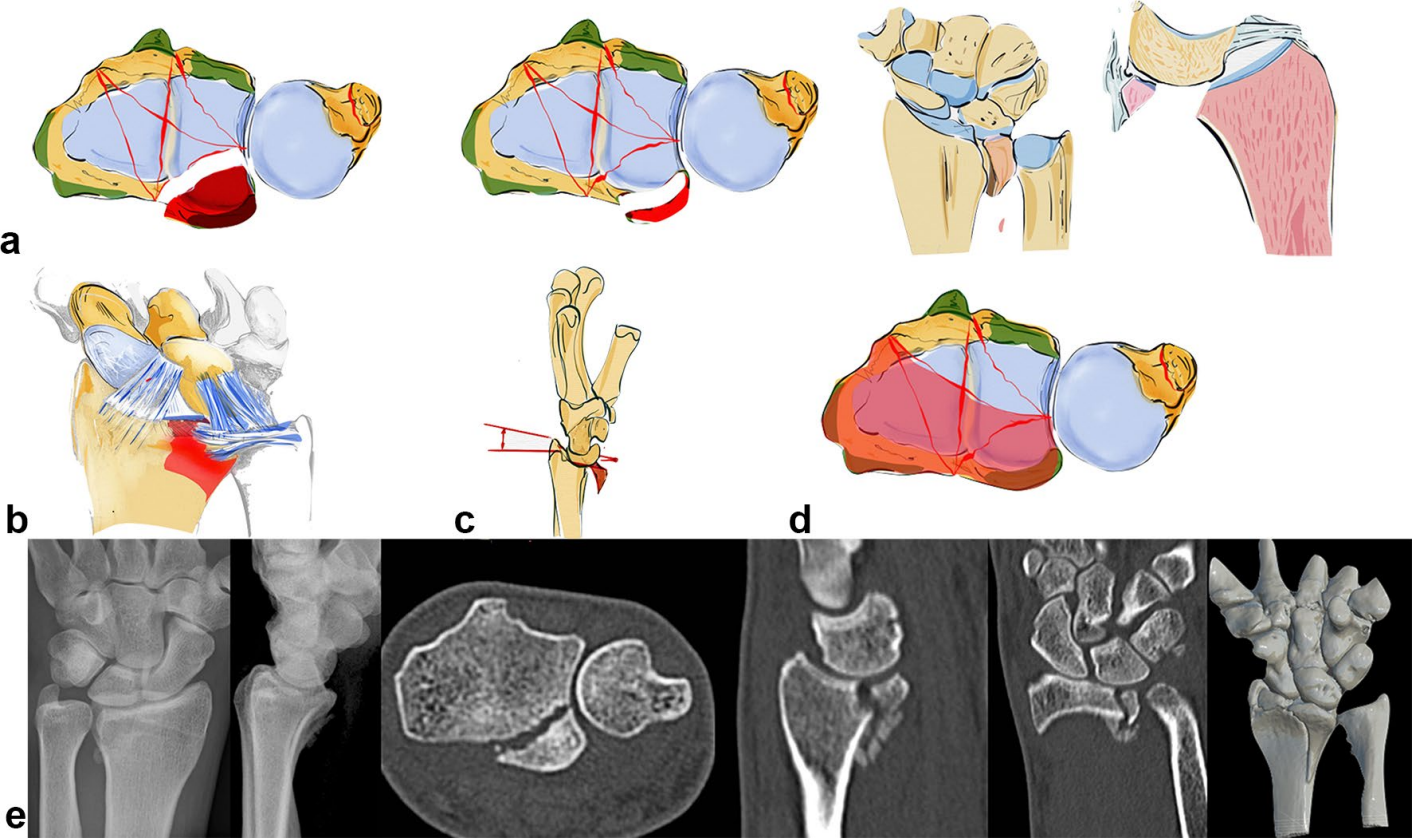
Palmar key type: the palmar ulnar osteoligamentous unit can either be a large or smaller rim fragment. Dislocation can occur in a palmar direction with the dorsal ligaments remaining intact (a). Palmar ulnar fragment: origin of important radioulnar and ulnocarpal ligaments (b). The osteoligamentous unit dislocates in a palmar direction (c). In extreme cases, a complete ulnar to radial palmar fragment is possible (d). Small ulnar palmar fragments can be easily overlooked on plain X-rays, axial CT scans however show this fragment best (e)
